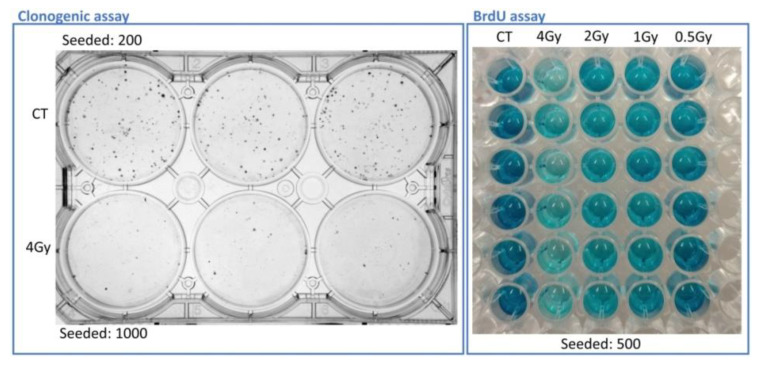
A375 plates for both clonogenic and proliferative assays. Left, plate for clonogenic assay with the control sample (CT) and the sample irradiated with a neutron dose of 4 Gy at day seven after irradiation. Right, BrdU cell proliferation assay of control and irradiated cells at day four after irradiation. The samples were analyzed in triplicate for each data point.
